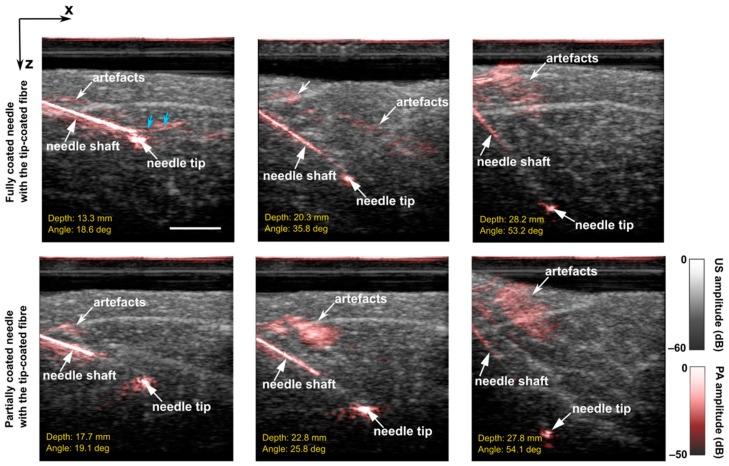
Overlaid Photoacoustic (PA) and Ultrasound (US) images acquired during in-plane needle insertions into a pork joint tissue ex vivo with a fully coated needle and a partially coated needle (tip-coated fibres inside the lumens). Blue arrows denote arc-shaped artefacts from the needle tip. Images were obtained from real-time reconstructions and displayed on a logarithmic scale. All the images have the same scale bar of 1 cm.
